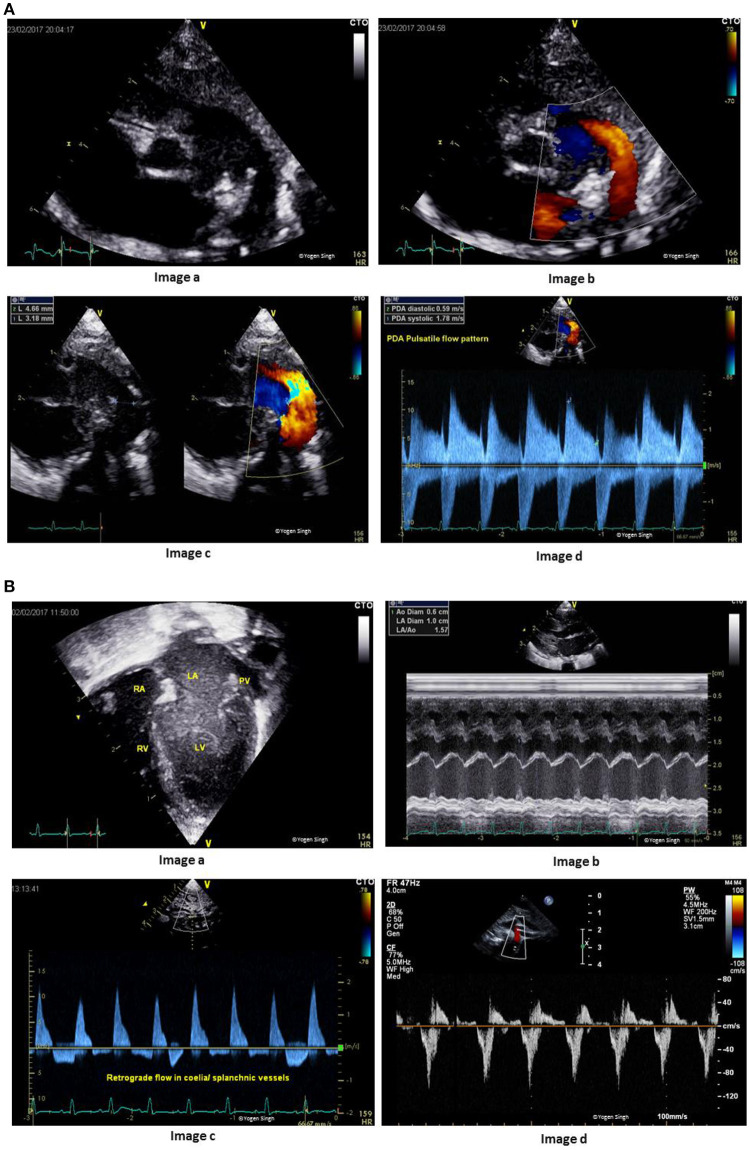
(A) Assessment of patent ductus arteriosus (PDA). Image “a” shows PDA on 2D imaging; Image “b” shows PDA on color flow mapping; Image “c” assessment of PDA size on 2D and color flow mapping; and Image “d” shows pulsatile flow pattern on Doppler assessment. (B) Hemodynamic assessment of PDA for assessing pulmonary hyperperfusion and systemic hypoperfusion. Image “a” shows volume overloading left side of the heart in apical 4-chamber view; Image “b” shows measurement of left atrium to aorta ratio (LA:Ao ratio); Image “c” shows retrograde flow in splanchnic vessels; and Image “d” retrograde flow in descending aorta (“steal phenomenon”) in a hemodynamically significant large PDA.
